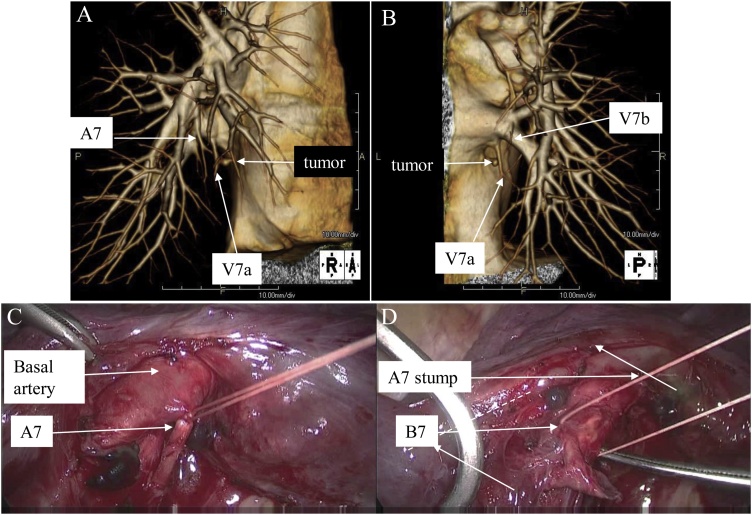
Three-dimensional CT and intraoperative findings (A) Three-dimensional CT in the right anterior oblique view showing the location of the tumor and the pulmonary vessels. (B) Three-dimensional CT in the posterior view shows V7b (marking the intersegmental plane between S7 and S10). (C)A7 branching from the basal artery was ligated. (D) B7 was isolated from behind A7.
